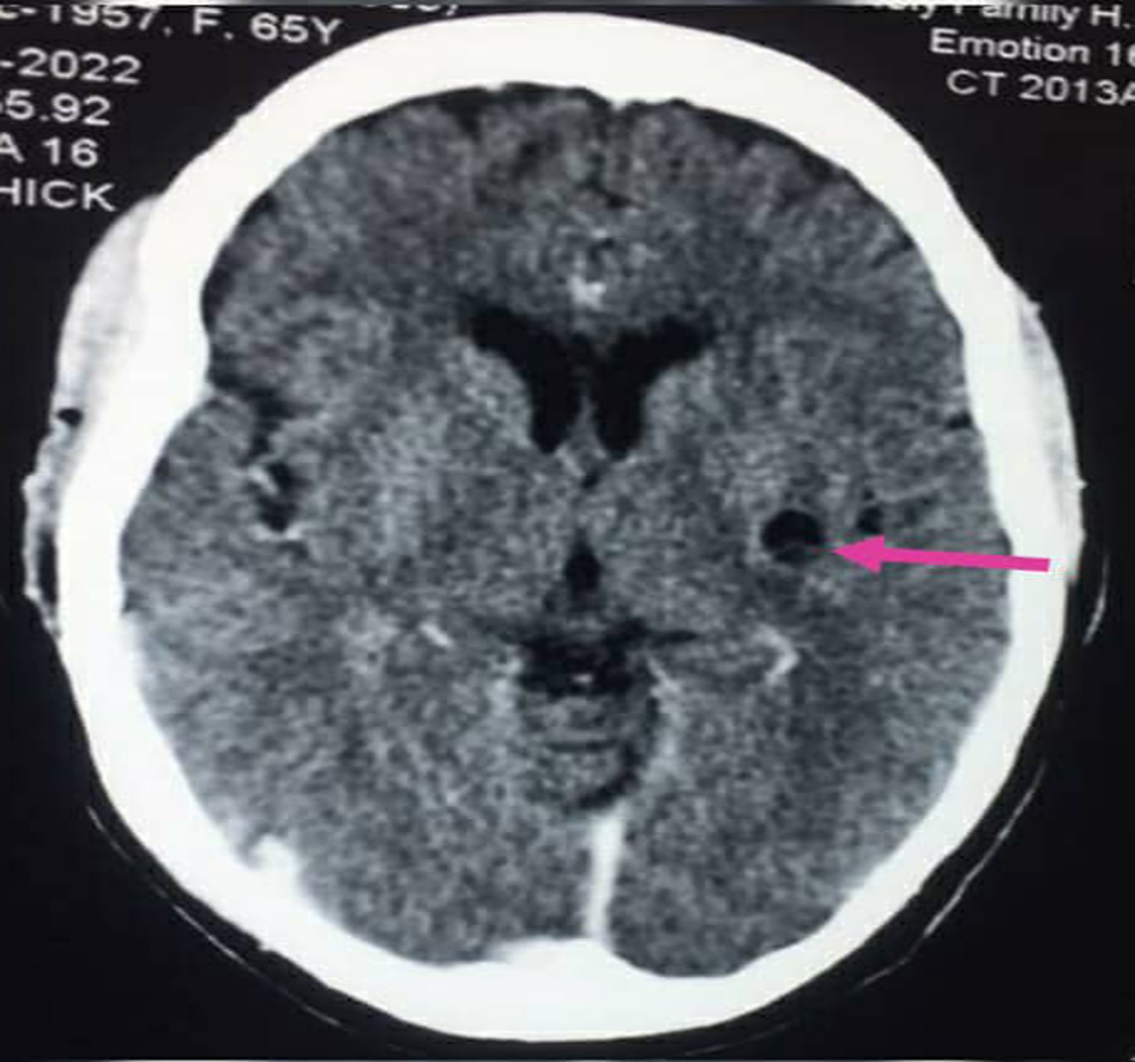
Contrast enhanced axial computed tomography (CT) scan image of the brain showing cyst with scolex in the left insular cortex.
